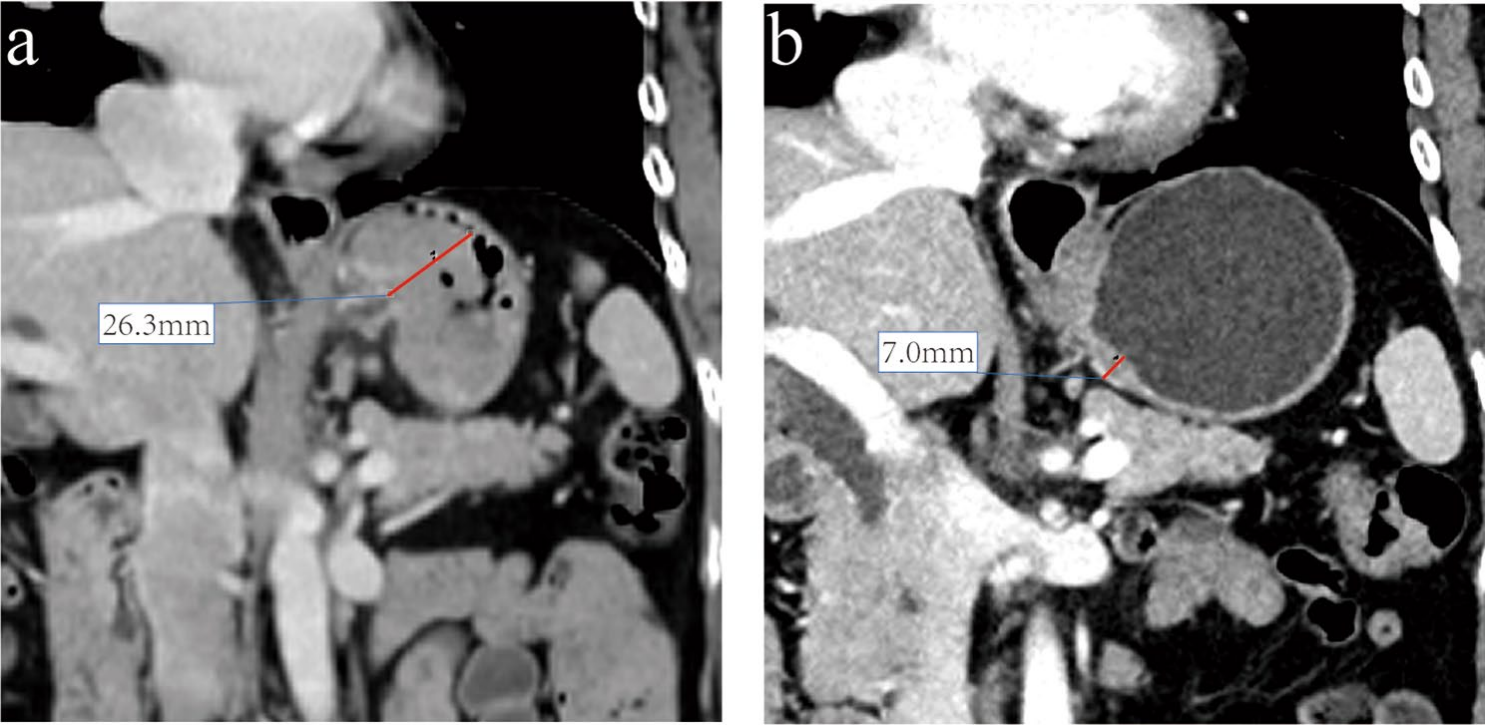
Female, 63 years, gastroscopy revealed a mass at the gastroesophageal junction. The biopsy pathology showed moderately differentiated adenocarcinoma with intestinal type of Lauren classification. The clinical stage was diagnosed as cT4aN1 by the preoperative CT examination. This patient received 3 cycles of XELOX chemotherapy and total gastrectomy. Postoperative pathology showed that the residual carcinoma in the original tumor bed was less than fibrous tissue hyperplasia. No metastases were observed in lymph nodes dissected in surgery (0/51). A: the baseline coronal CT image showed the gastric wall was significantly thickened. B: the coronal CT image after chemotherapy showed the primary tumor thickness shrunk
